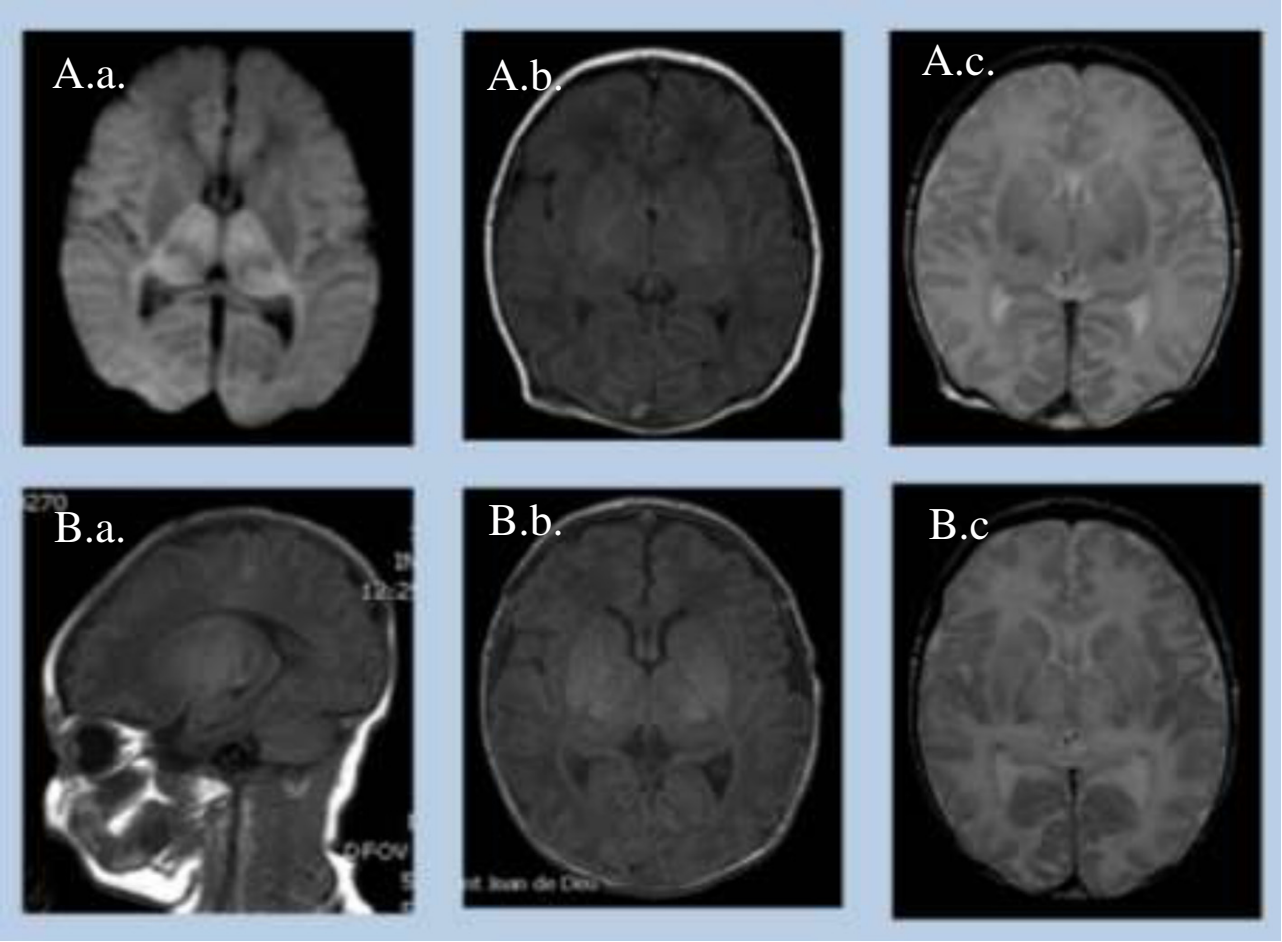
Sequential MR studies in a newborn with severe HIE and a central pattern of injury (ID number 12). A. Early MR study. A.a. Axial diffusion-weighted image: restricted diffusion in the thalami; A.b. Axial T1 weighted images: abnormal high intensity within the basal ganglia and thalami (BGT) and loss of the normal intensity of the posterior limb of the internal capsule. A.c. Axial T2 weighted image. Low and high signal intensity within the basal ganglia and thalami. B. Late MRI study confirmed the previous findings. Sagittal (B.a.) and axial (B.b.) T1 weighted images showing an abnormal widespread high intensity of BGT and confirming the absence of the normal intensity of the posterior limb of the internal capsule. Cortical highlighting around central fissure. B.c. Axial T2 weighted images with heterogeneity of the signal in BGT more evident than in the early MRI.
